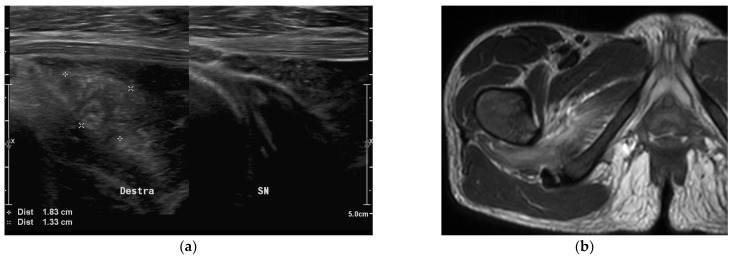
Pyomyositis of the quadratus femoris muscle in a 15-year-old girl affected by end-stage renal disease. (a) Soft tissue ultrasound shows enlargement and inhomogeneous hyperechogenic texture of the right muscle, compared to the healthy contralateral muscle. (b) Axial T2-weighted MR image shows hyperintense signal in the muscle, no bone or joint involvement.
